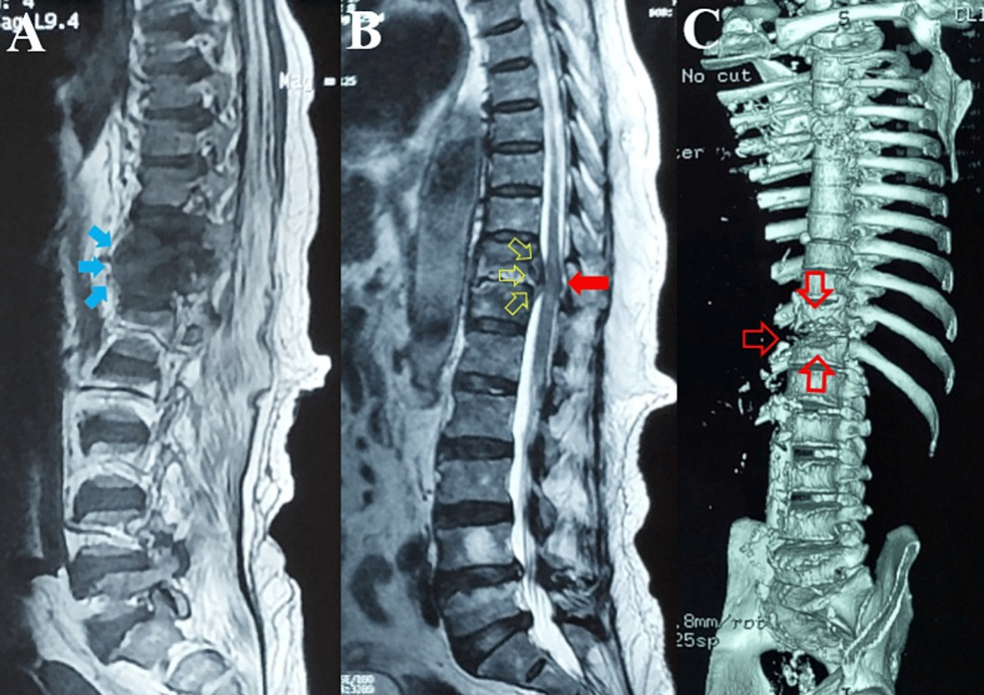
(A) T1 weighted Sagittal Spine MRI showing a pre and paravertebral abscess at the D11-D12 level (blue filled arrows). (B) T2 weighted Sagittal Spine MRI showing disk involvement at the D11-D12 level with a posterior wall abscess (hollow yellow arrows) and spinal cord compression (red filled arrow). (C) Computed tomography 3D reconstruction of the spine showing bone loss at the D11-D12 level (hollow red arrows).
